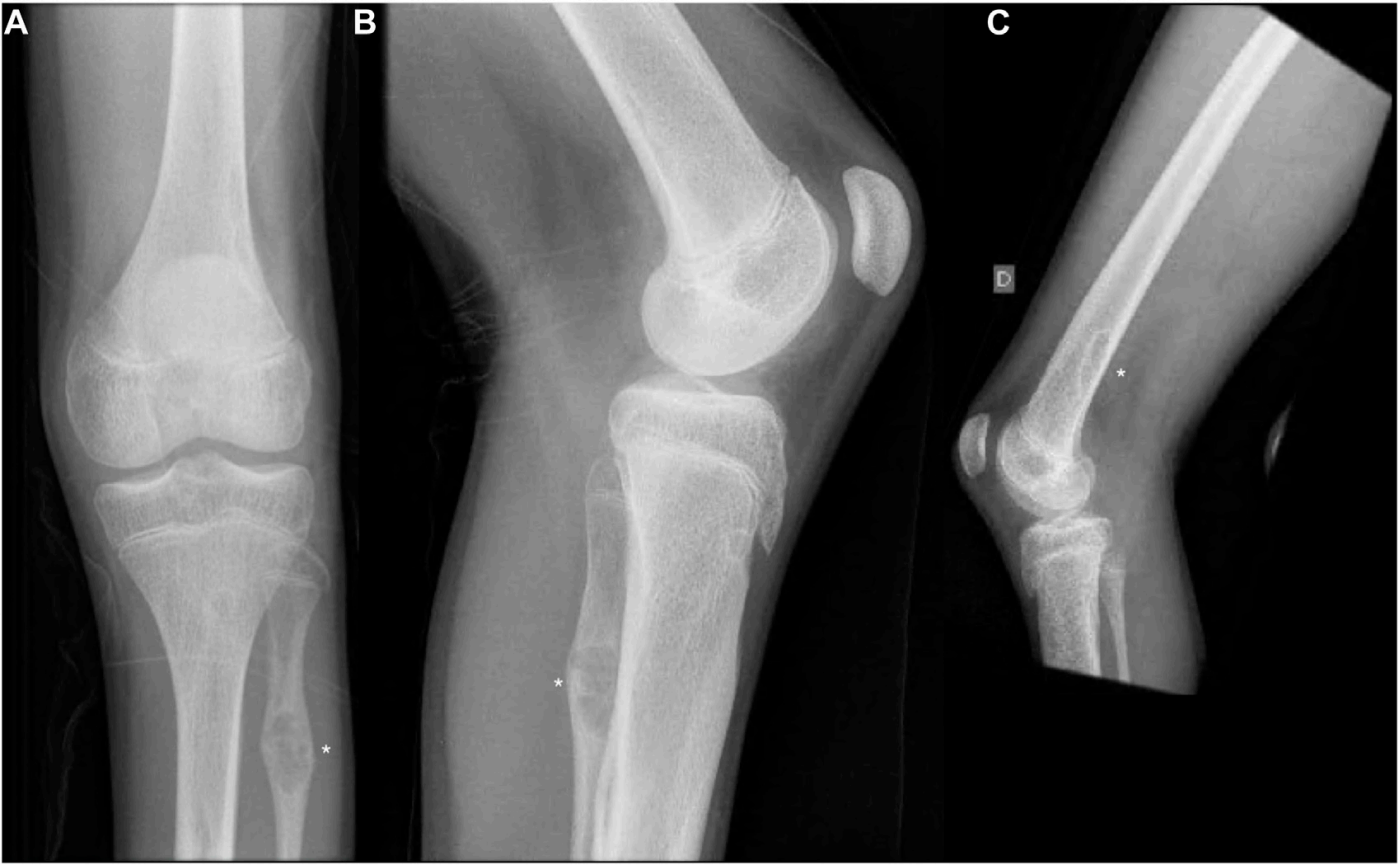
(A,B) Anteroposterior and lateral radiographs of the left knee showing non-ossifying fibroma in the distal femur and proximal fibula. (C) Lateral radiographs of the right knee showing non-ossifying fibroma in the distal femur. Non-ossifying fibromas are indicated by asterisks.
